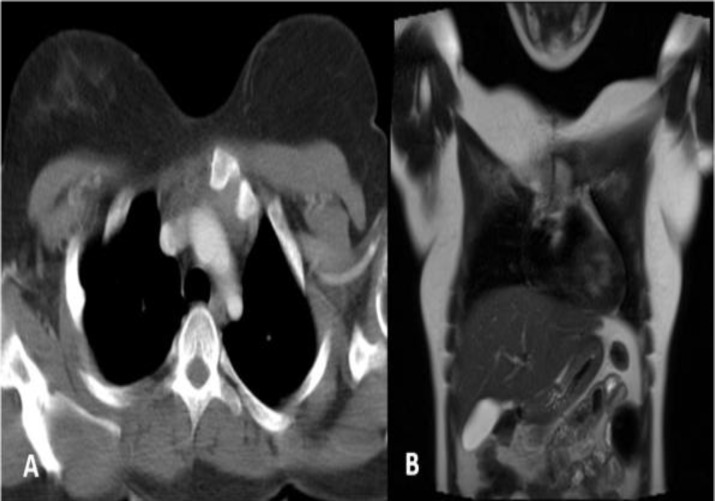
Post operative CT and MR images of the patients. There is no evidence of tumoral remnant in bed of right clavicular resection in axial CT (A) and coronal T2-weighted (B) images.
